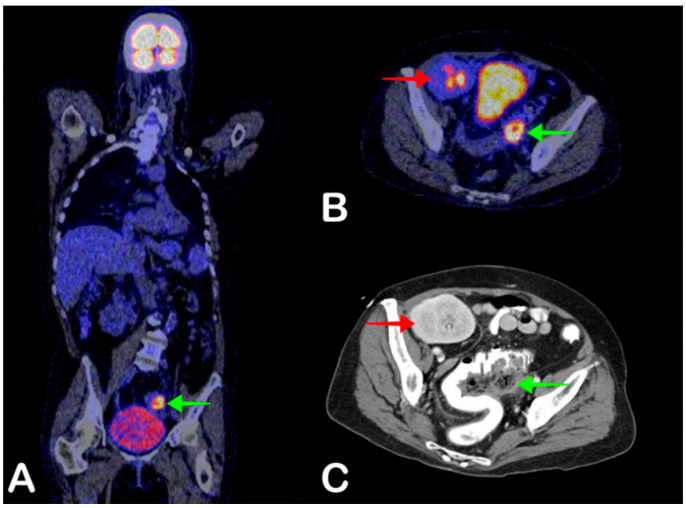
A 54-year-old woman was admitted to the hospital with fever. During hospitalization, she repeatedly had blood cultures positive for *Escherichia coli* and *Klebsiella pneumoniae* without localizing symptoms. Two years earlier, she had received a kidney transplant. Fused coronal2-deoxy-2-[18F]fluoro-D-glucose Positron Emission Tomography combined with Positron Tomography (FDG-PET/CT) showed increased FDG uptake at the left side of the sigmoid colon (A, green arrow), also shown on fused axial FDG-PET/CT (B, green arrow), indicative of either diverticulitis or abscess formation, as an explanation for the positive blood culture (*Escherichia coli*). Antibiotic treatment was started, but 9 days after PET/CT, the patient developed severe abdominal pain, and abdominal CT was performed. Axial CT also shows the abscess, with adjacent free intraperitoneal air, in keeping with perforated diverticulitis (C, green arrow). The kidney transplant in the right iliac fossa is also visible (B and C, red arrow). A laparotomic sigmoidectomy was performed, after which the patient recovered.
